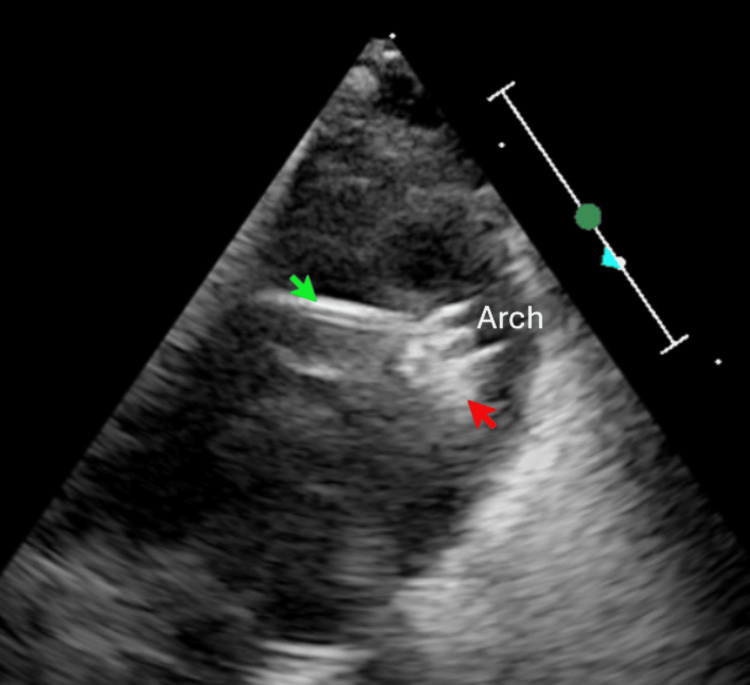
Echocardiography demonstrates the device (indicated by the red arrow) loaded within the delivery sheath (green arrow) and positioned at the patent ductus arteriosus (PDA).
